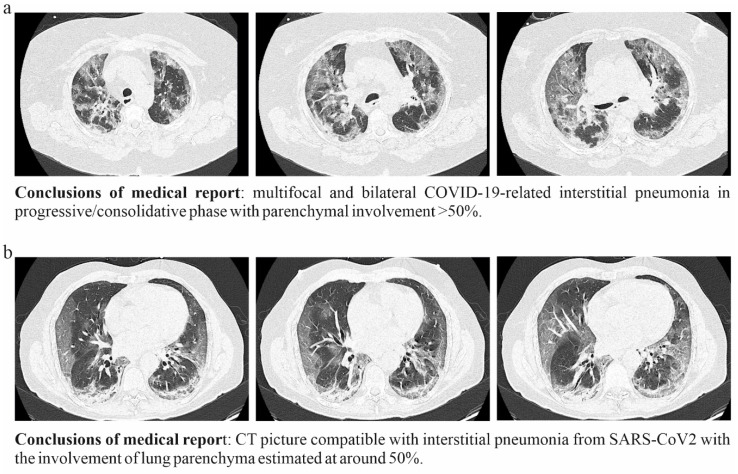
CT lung scan pictures of two randomly chosen COVID-19 patients (a,b) enrolled in the study.
